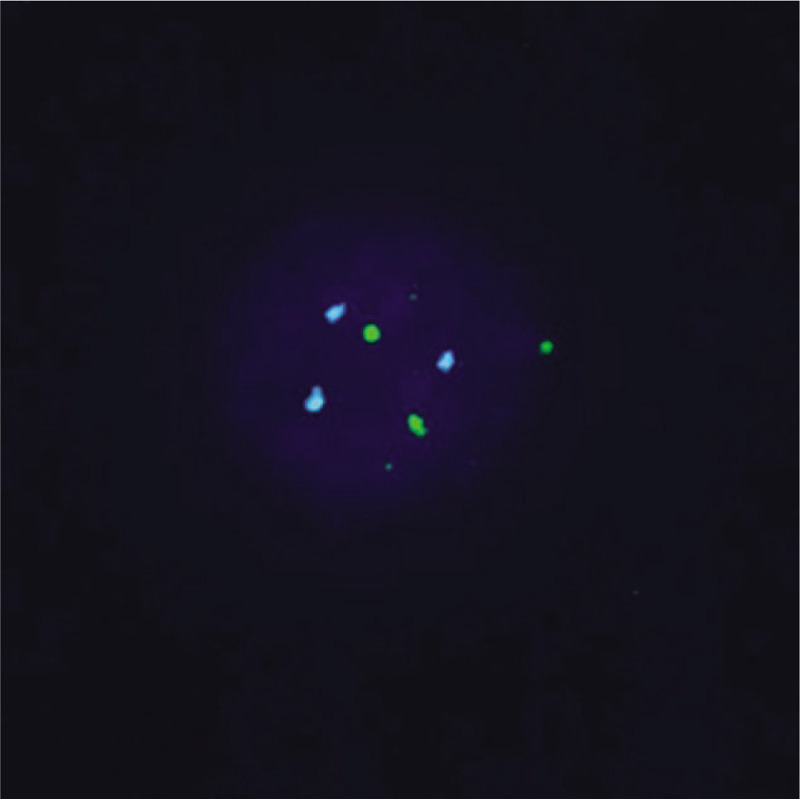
Abnormal sonographic features of fetus at 23th week of gestation. A: Overriding fingers. B: Atrioventricular septal defect.
